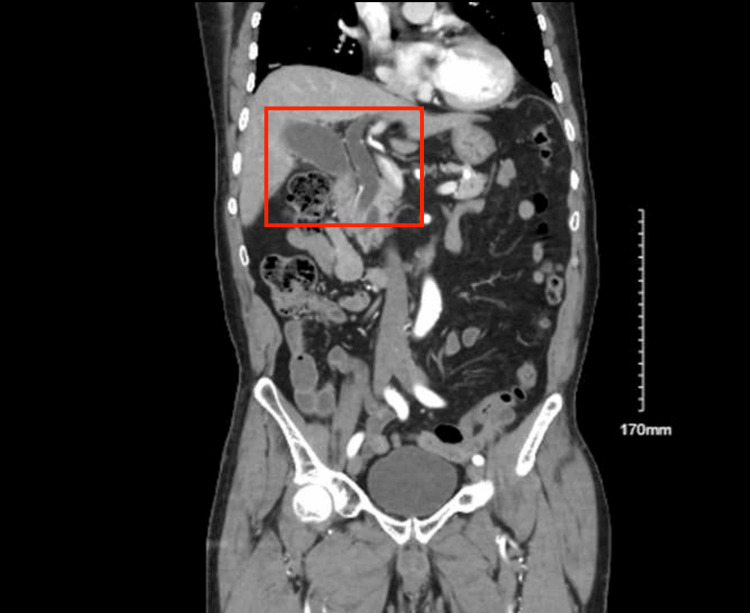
CT scan (coronal section) showing common biliary duct and pancreatic duct dilatation (~12 mm and 2 mm, respectively), secondary to periampullary diverticulitis-induced double-duct sign.
